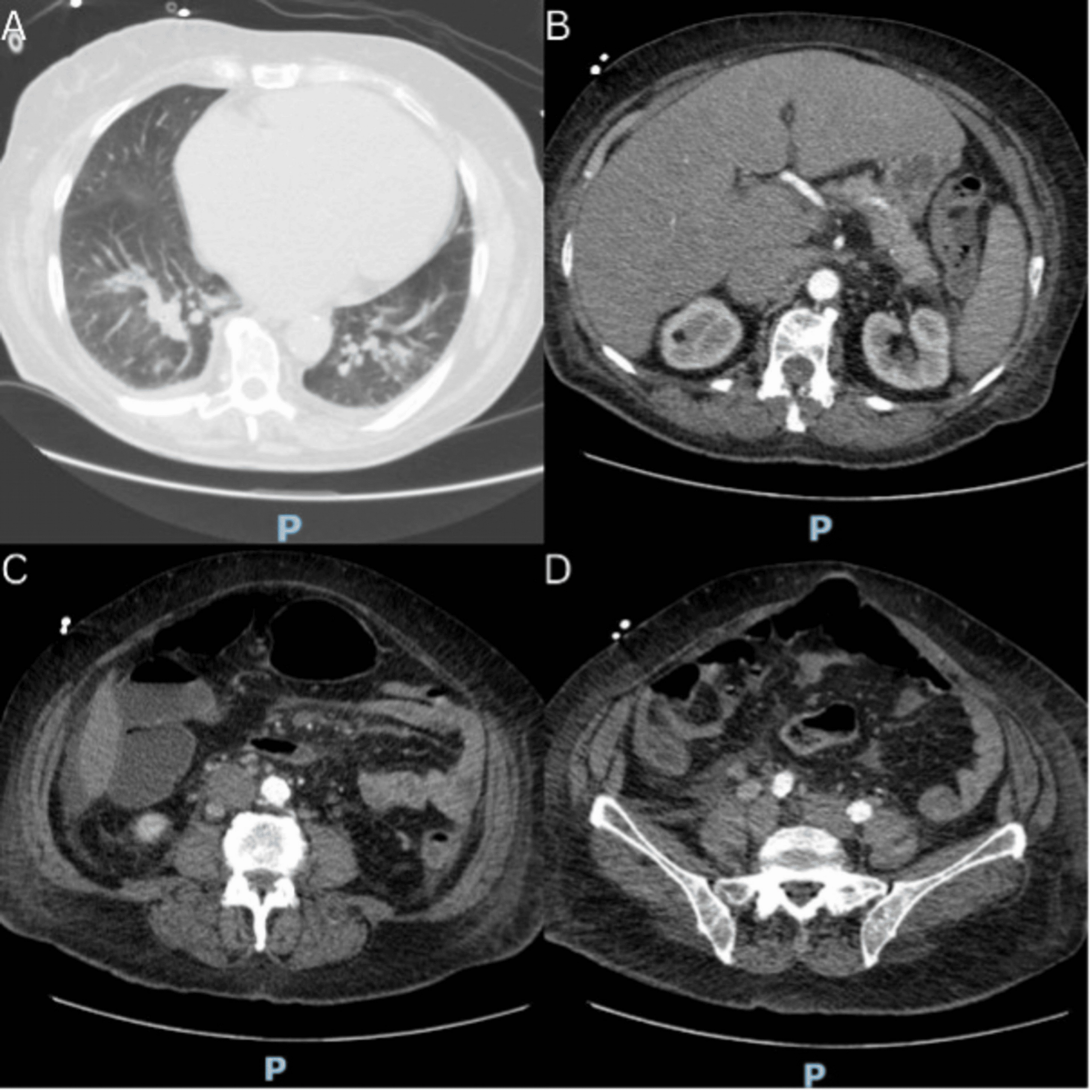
Full-body CT scan at different levels (A–D) A) Severe cardiomegaly and mild pleural effusion on the right (with 2 mm of thickness). B) Hepatomegaly; the liver measures 20 cm at the midclavicular line and features generalised reduction of its density. Dilatation of the retrohepatic inferior vena cava and of the suprahepatic veins reveals reflux of the contrast to the suprahepatic veins during the arterial phase. Mild splenomegaly (spleen measuring at 13.2 cm). C–D) Mild dilatation of the inferior vena cava. Multiple latero-aortic adenopathy is seen (the largest with a 1.3 cm diameter) in addition to some adenopathy in the common iliac chain (the largest is 1.1 cm) and in the inguinal region (the largest is 1.7 cm).
